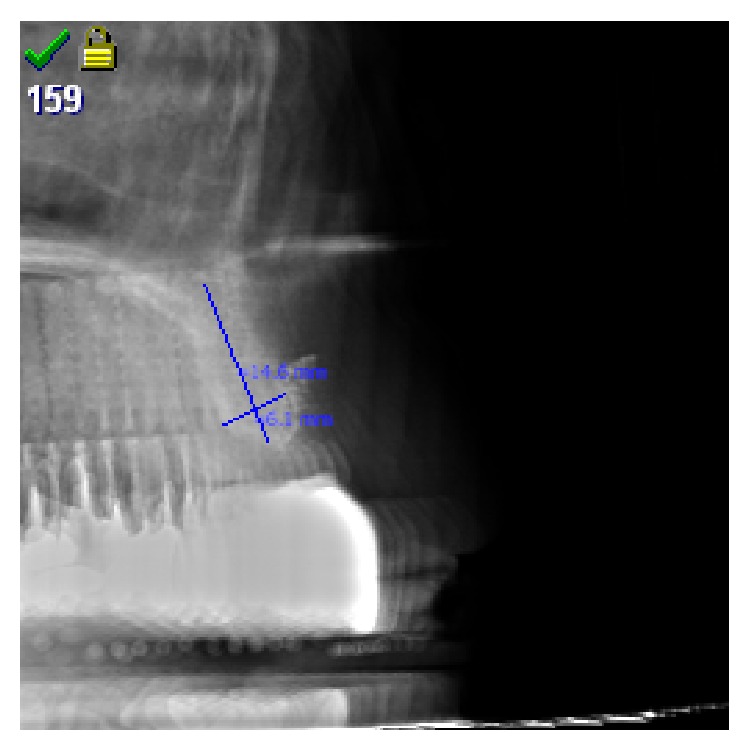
CBCT control and measurement of achieved bone dimensions prior to implant insertion.
